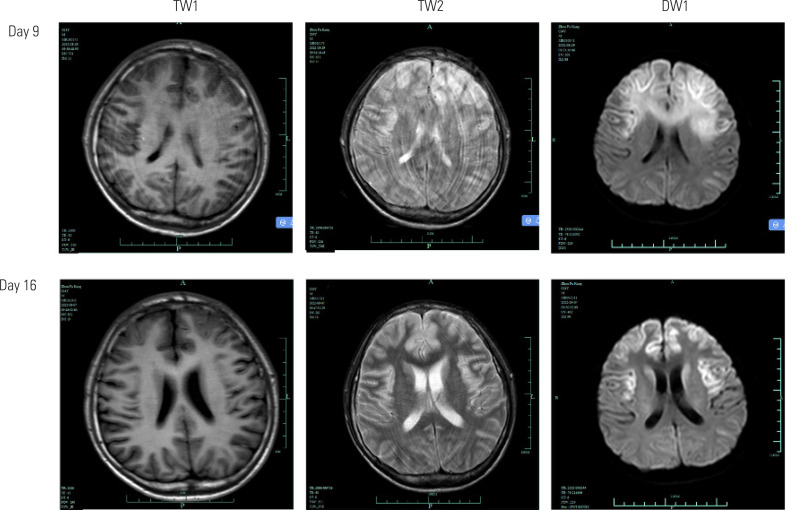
Results of magnetic resonance imaging (MRI) scans obtained on the 9th and 16th day of admission. Abbreviations – T1: T1-weighted sequences; T2: T2-weighted sequences; DWI: diffusion-weighted sequences.
